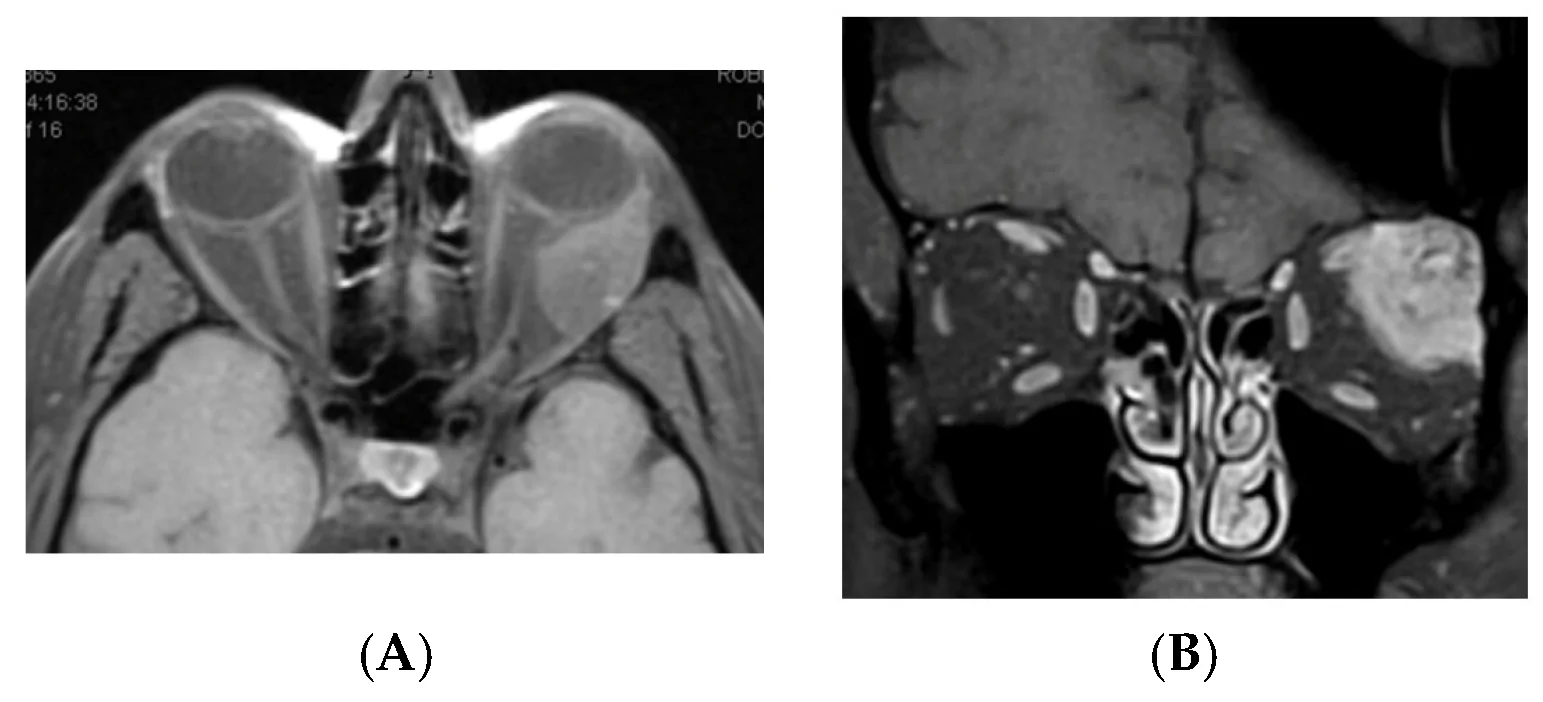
A 60-year-old man presented with 6 months history of progressive painless proptosis of the left eye. (A,B) MRI axial (A) and coronal (B) T1 weighted post-contrast fat-suppressed images show a left lacrimal gland mass. An incisional biopsy confirmed lacrimal gland ACC. The patient subsequently had eye-sparing gross total resection of the left lacrimal gland mass followed by adjuvant radiation therapy.
